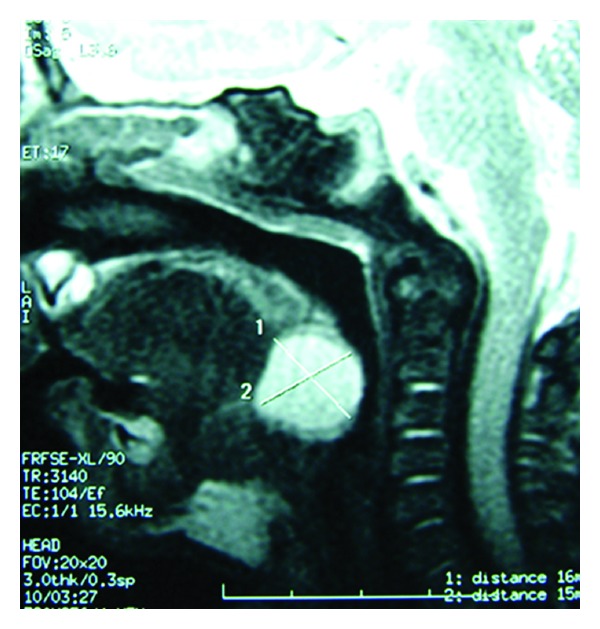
MRI in sagittal view shows a large cystic mass at the tongue base.
